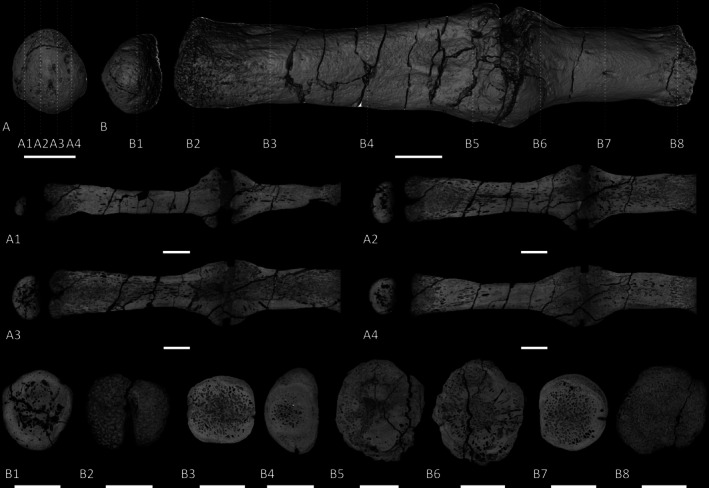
CT scan of *Barosaurus* sp. NAMAL‐106 terminal two caudal vertebrae CaA and CaB. (A, B) 3D rendering (A) CaB posterior view, (B) CaA left lateral view and CaB right lateral view; (A1–A4) CT scan slices in parasagittal cross‐section, (B1–B8) CT scan slices in transverse cross‐section. Scale bars = 10 mm.
